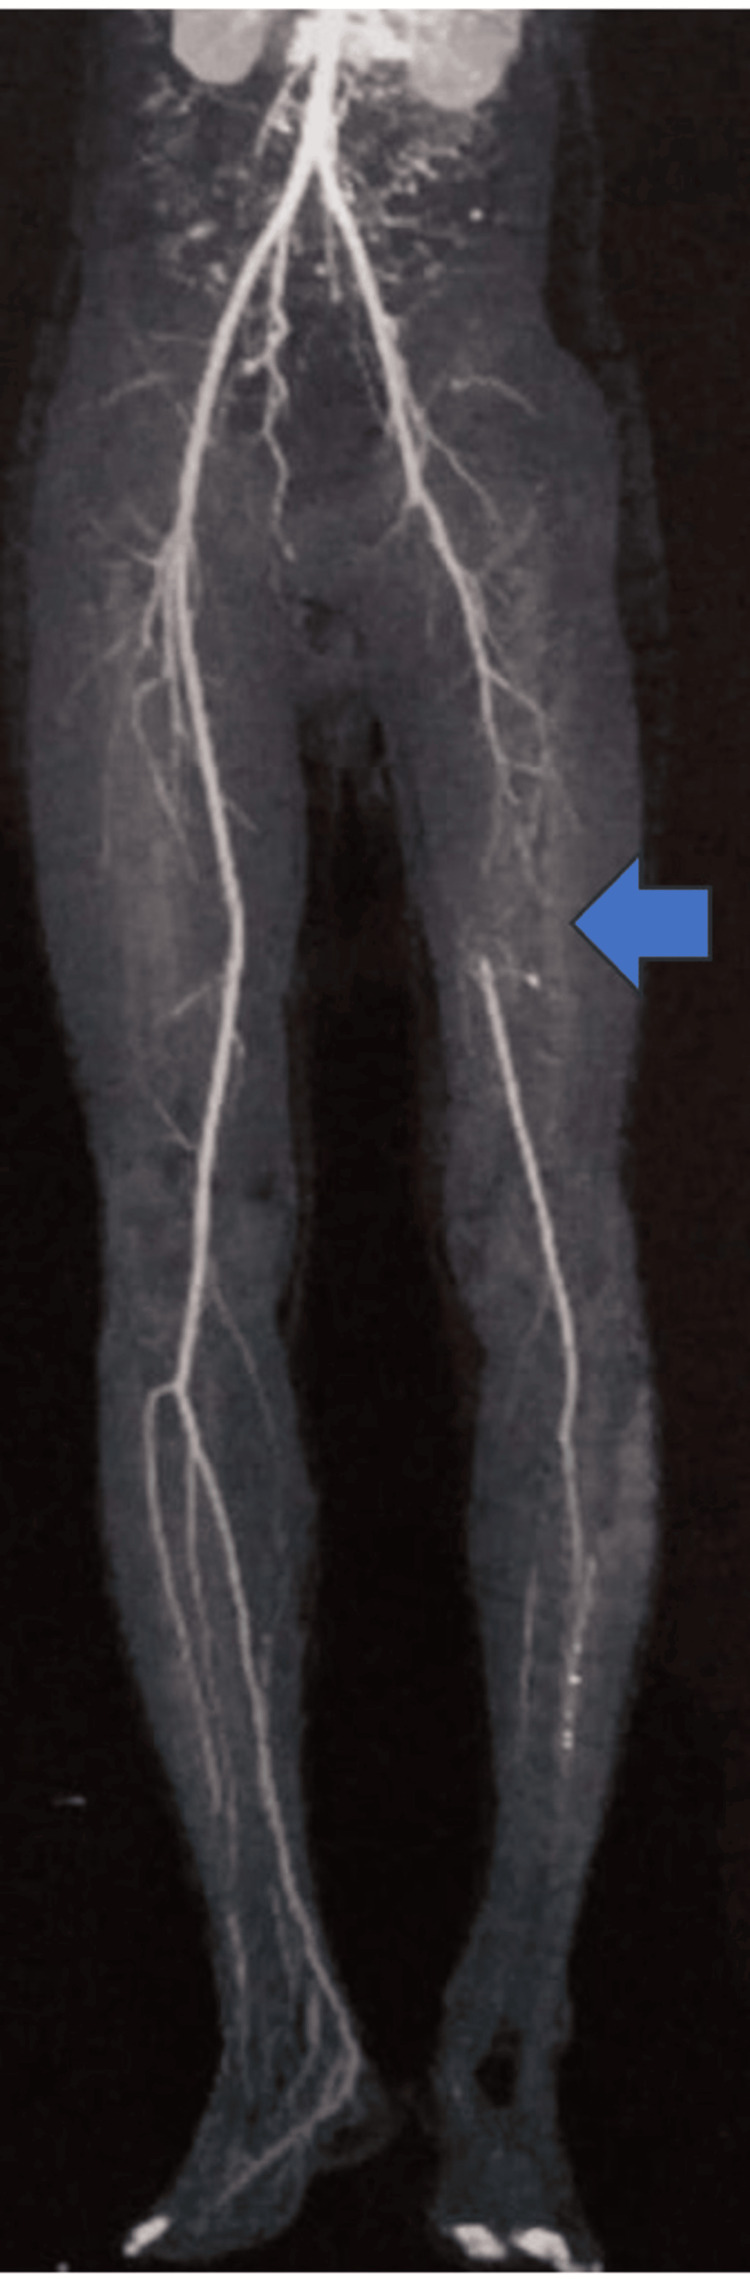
CT Angiogram Showing Long Segment Obstruction in the Left Superficial Femoral Artery with Collateral Formation (Arrow Showing No Contrast between Proximal and Distal Superficial Femoral Artery) CT: Computerized Tomography
